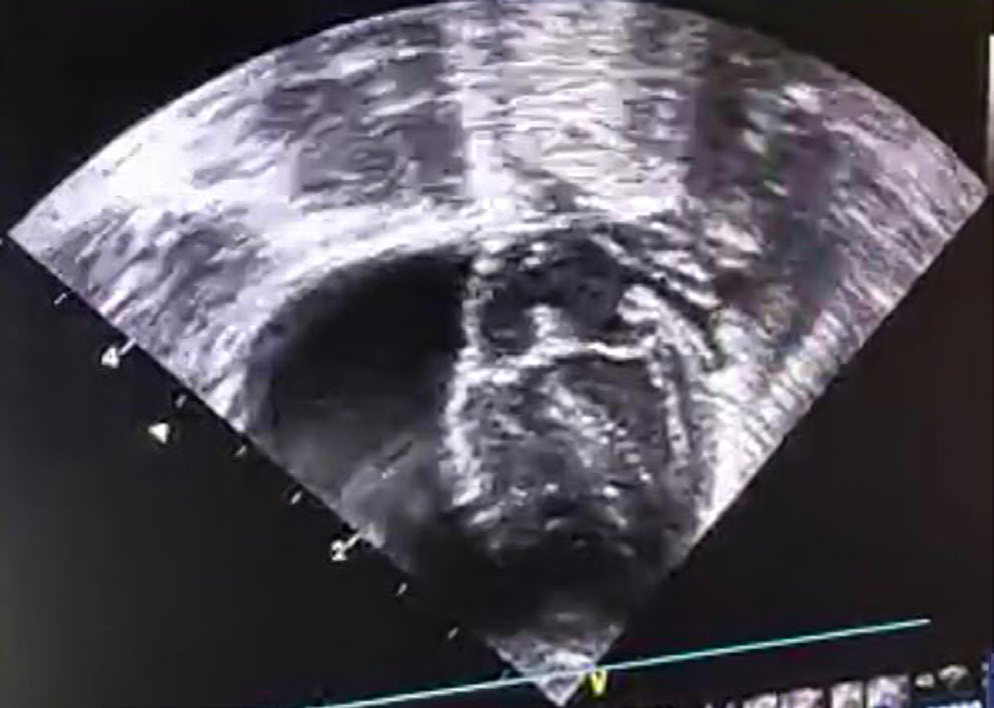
Echocardiography shows the huge pericardial effusion
